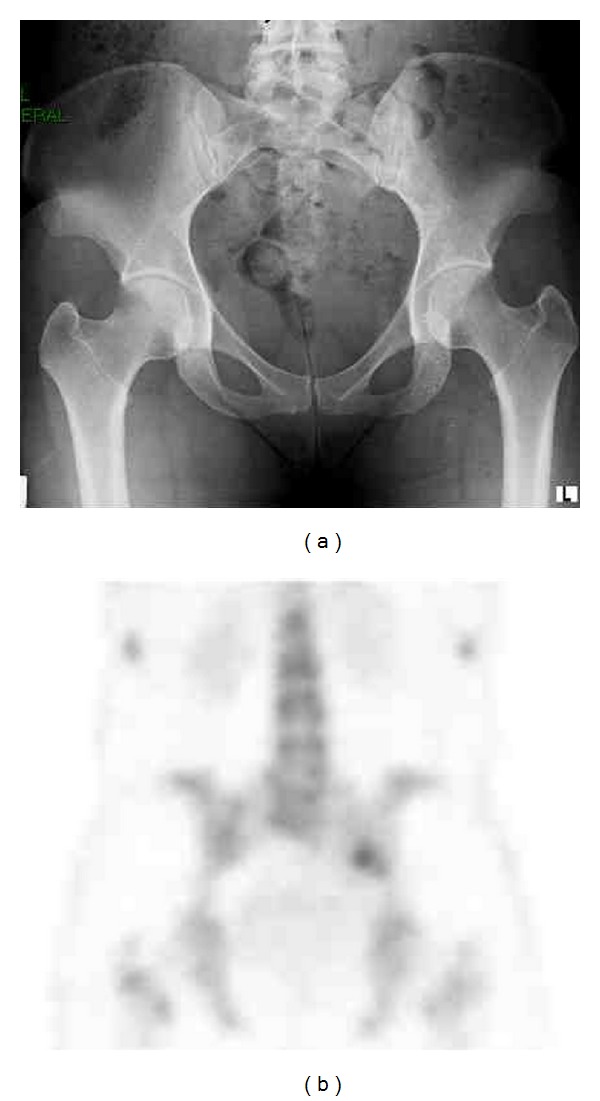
Imaging of the patient at 18 months after surgery: (a) pelvic X-ray shows mild narrowing of the left hip joint and (b) Tc-99m bone scan shows mild uptake at the left sacroiliac joint.
